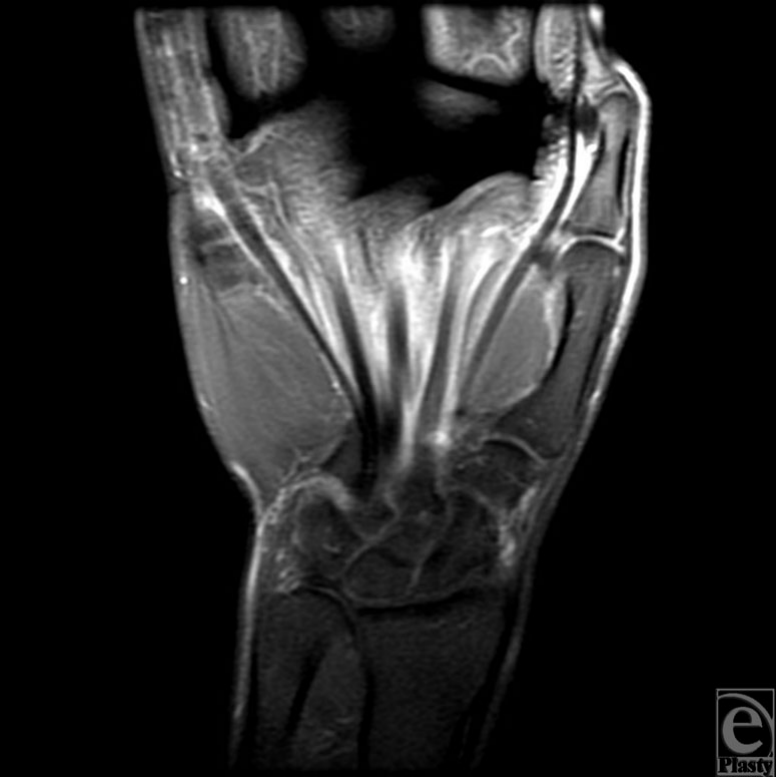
Coronal post contrast fat-suppressed T1-weighted MRI demonstrates communication between the common flexor tendon sheath and the tendon sheath of the flexor pollicis longus tendon.
